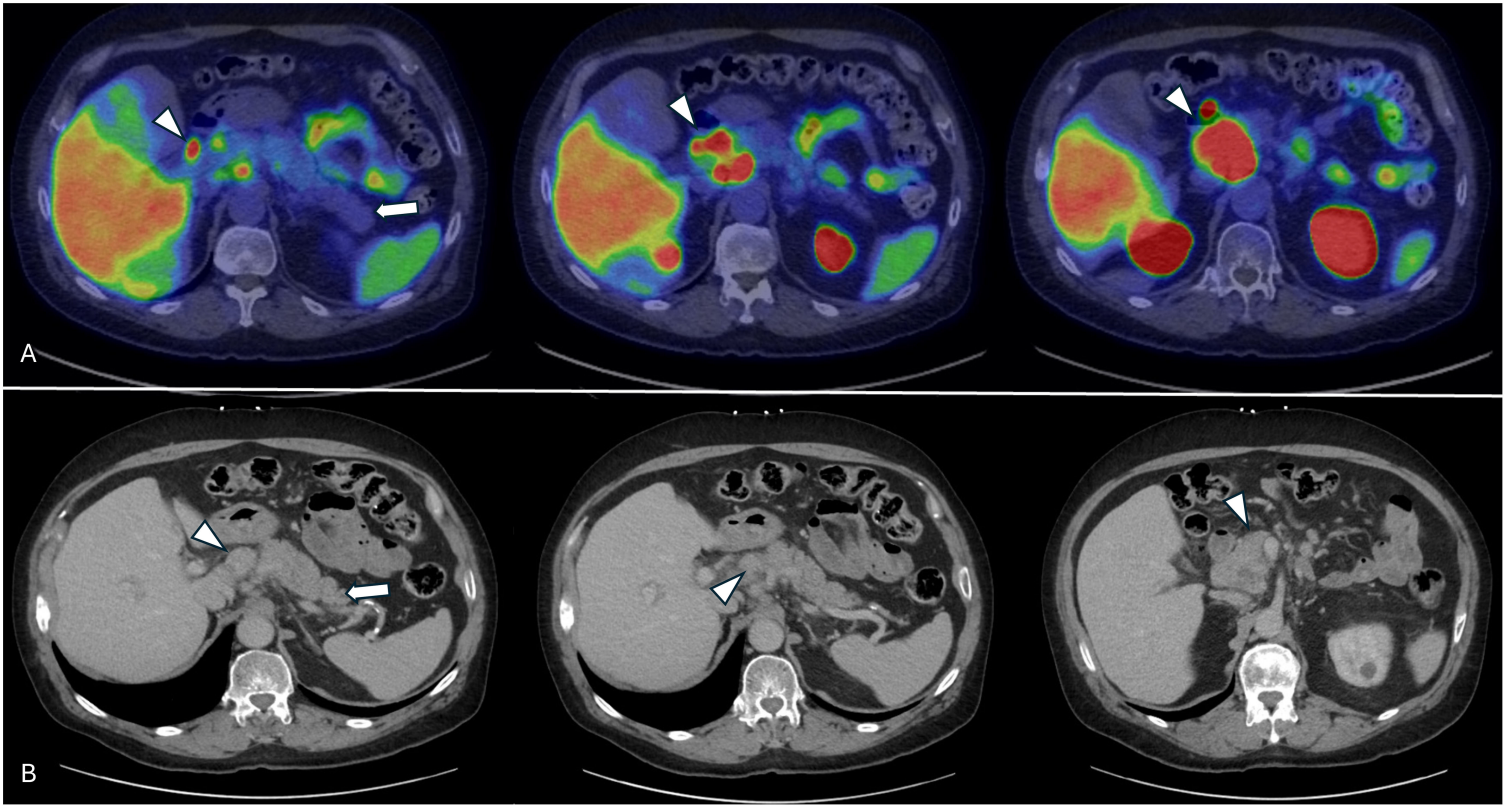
Pretherapy axial views of the pancreas (arrow) and peripancreatic metastatic lymph nodes (arrowheads) showing no inflammatory changes within the pancreas. (A) Axial views from the pre-therapy [^68^Ga] Ga-PSMA-11 PET/CT scan. (B) Pre-therapy contrast-enhanced CT images showing the pancreas and multiple peripancreatic metastatic lymph nodes.
